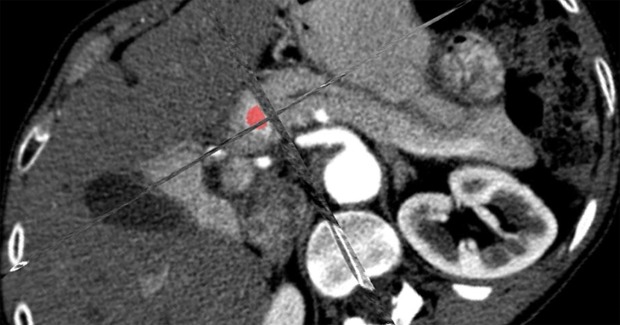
3D ROI within a small neuroendocrine isthmus pancreatic tumor on CT image on pancreatic phase for texture analysis.
